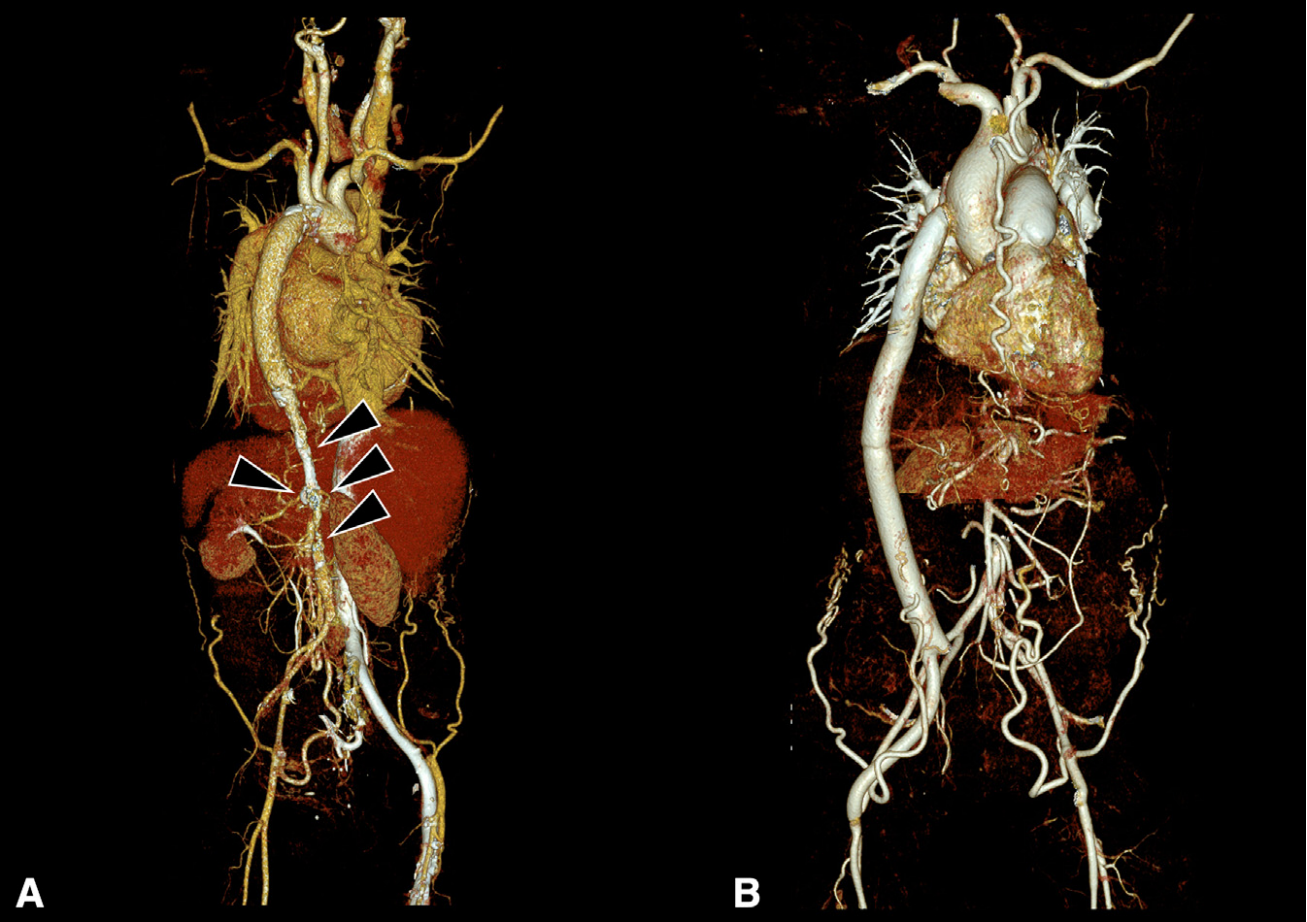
Three-dimensional computed tomography (*CT*) images. A, Posterior view of preoperative CT scan showing multifocal luminal narrowing of the descending thoracic aorta, abdominal aorta, and both renal arteries. B, Anterior view of postoperative CT scan showing a patent bypass graft.
